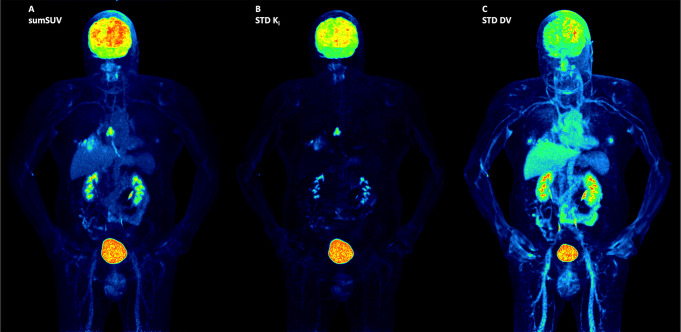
sumSUV, K_i_ and DV of STD Patlak MIP images from a lung cancer patient. The K_i_ images show a higher phosphorylation rate in the primary tumor in the right lung as compared to the radiation necrosis in the dorsal part of the right lung. DV images demonstrate a higher DV in the radiation necrosis than in the primary lung tumor
